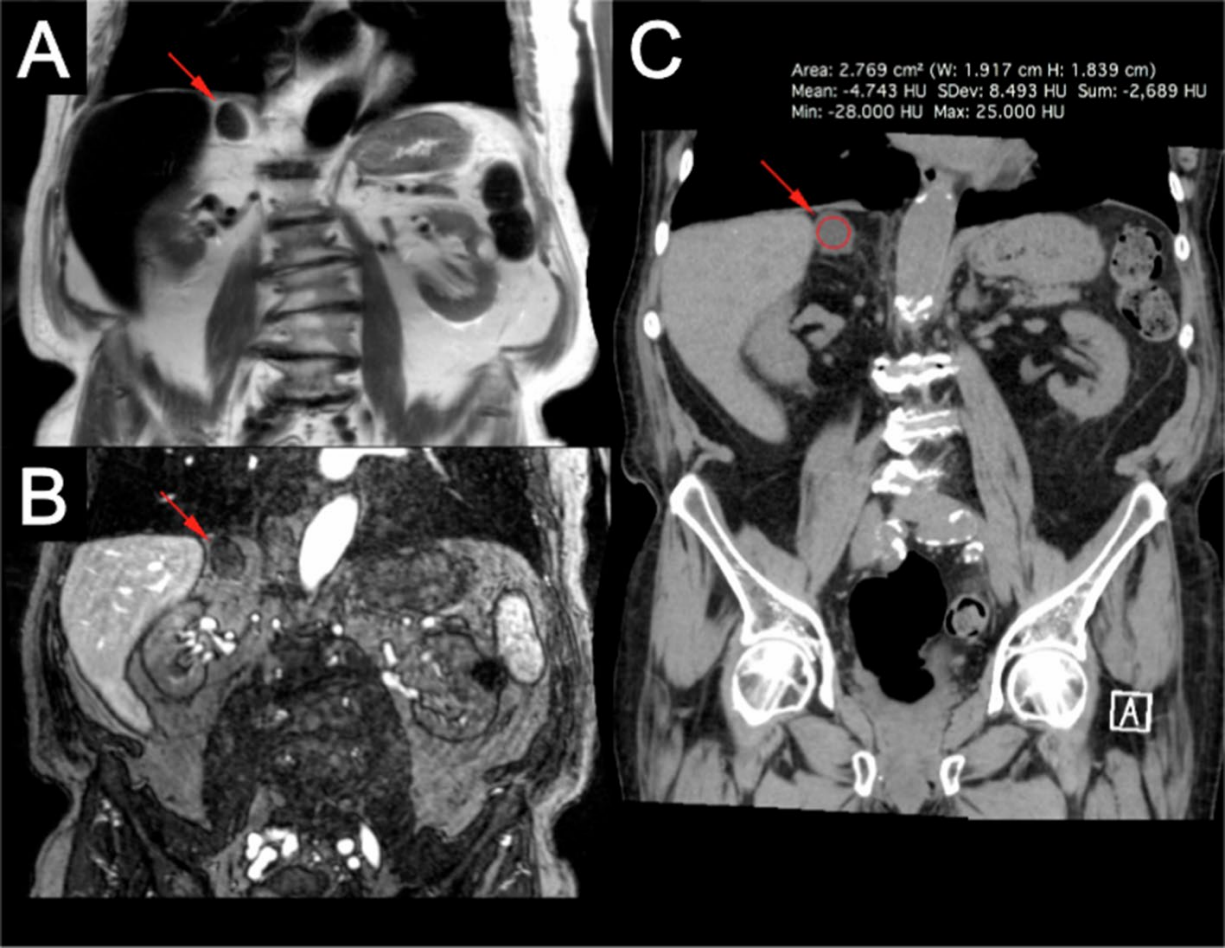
89-year-old male with a known adrenal adenoma. Post-contrast T2 (a) and T1 (b) 3.0 T ferumoxytol-enhanced MRI acquisitions show a solid enhancing adrenal lesion (red arrows). Follow-up non-contrast CT (C) confirms that the lesion is a lipid-rich adrenal adenoma (red arrow, red circle)
